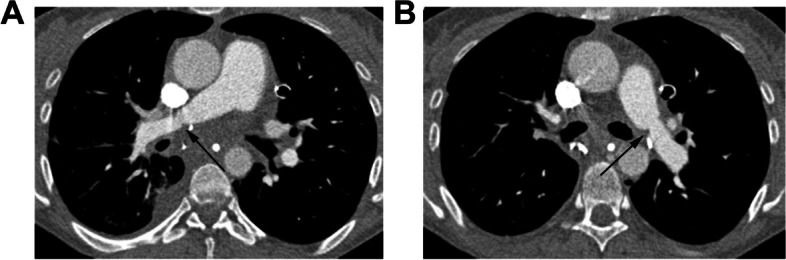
29-year-old female who underwent bilateral lung transplants for cystic fibrosis who developed pulmonary hypertension and pulmonary artery stenosis 3 weeks after surgery. A-B Axial contrast-enhanced CT images timed for the pulmonary arteries (A, B) show stenoses in the right (black arrow in A) and left (black arrow in B) pulmonary arterial anastomoses. Right heart catheterization showed elevated pulmonary artery pressures proximal to the stenoses with widened mean pressure gradients across the stenoses consistent with pulmonary hypertension. Stents were subsequently placed (not shown)
